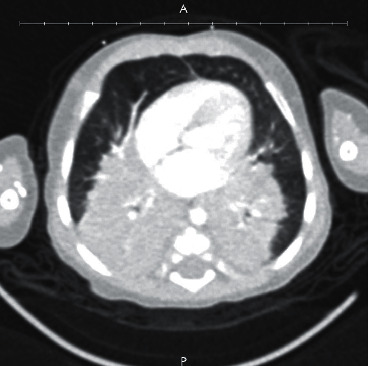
Computed tomography of the chest demonstrating large bilateral perihilar opacities at presentation.
